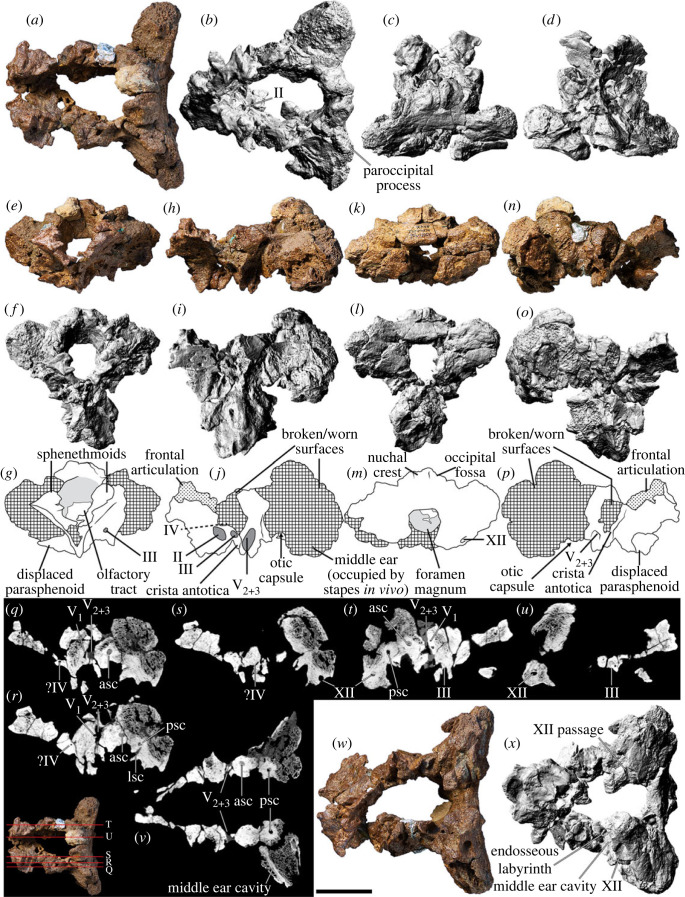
Diamantinasaurus matildae* referred braincase (AODF 0906). (*a–p*) Braincase in (*a*,*b*) dorsal, (*c*) posterodorsal, (*d*) anteroventral, (*e–g*) anterior, (*h–j*) left lateral, (*k–m*) posterior and (*n–p*) right lateral views. (*q–u*) Braincase cross-sections in (*q–s*) left lateral, (*t*,*u*) right lateral and (*v*) dorsal views. (*w–x*) Braincase in ventral view. (*a*), (*e*), (*h*), (*k*), (*n*) and (*w*) are photographs; (*b–d*), (*f*), (*i*), (*l*), (*o*) and (*x*) are three-dimensional models derived from surface scans; and (*q–v*) are CT slices. Scale bar = 50 mm.
